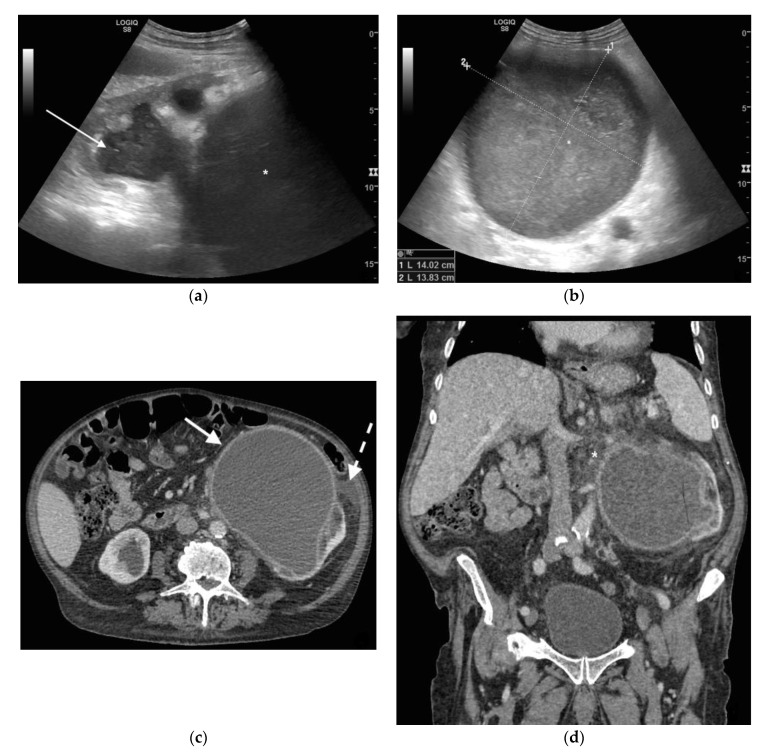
A 78 years old septic male patient. Ultrasound (US) longitudinal (a) and axial (b) view of the left kidney showing high-grade hydronephrosis with gross dilatation of the renal pelvis and calyces, filled by inhomogeneous urine (white arrow) and an extremely dilated inferior calyceal group filled by a huge ball of debris conglomerate (*). Axial (c) and MPR coronal Computed Tomography (CT) (d) after intravenous contrast in cortical-medullary phase, showing high grade hydronephrosis, diffuse parietal thickening of the calico-pelvic system (white arrow), fat stranding of perirenal and renal sinus fat. Small amount of free fluid is appreciated anteriorly (dashed arrow).
